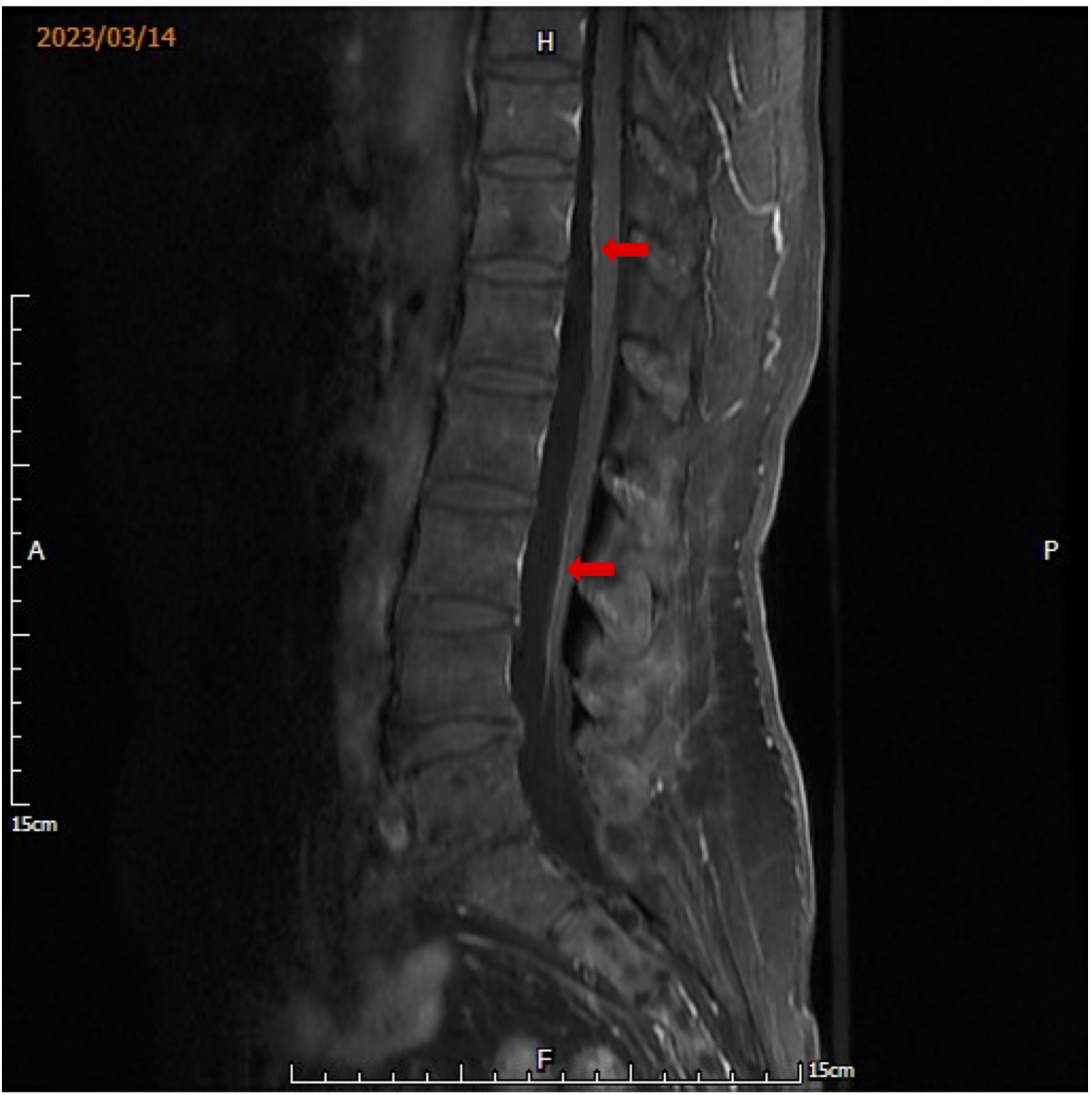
Whole spinal cord MRI showing multiple foci of striated enhancement of the spinal nerves, assessing metastasis in the soft spine (arrows are foci of spinal nerve enhancement).
